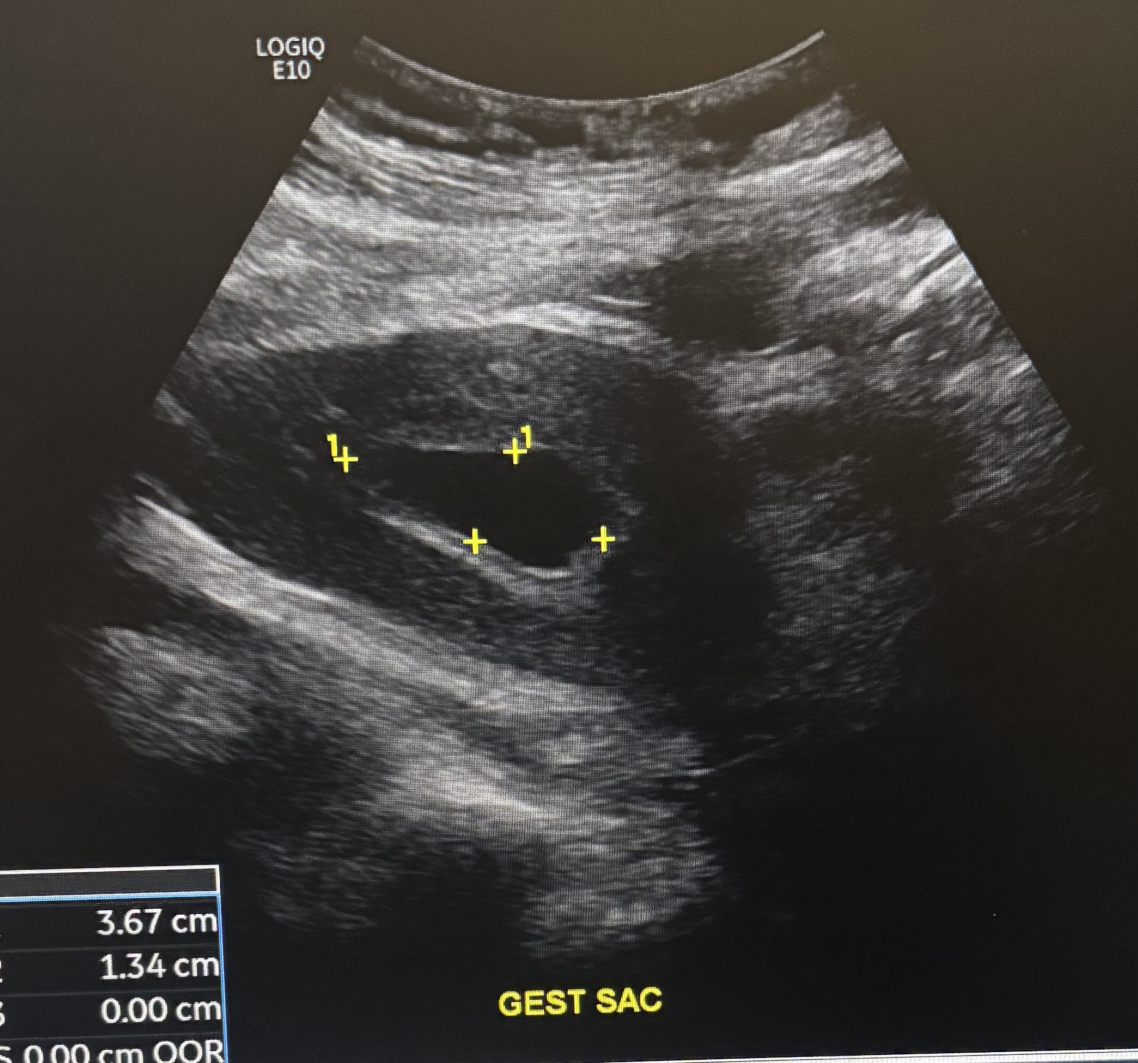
Ultrasound image of intrauterine pregnancy Ultrasound showing an intrauterine pregnancy with the gestational sac indicated by yellow markers
